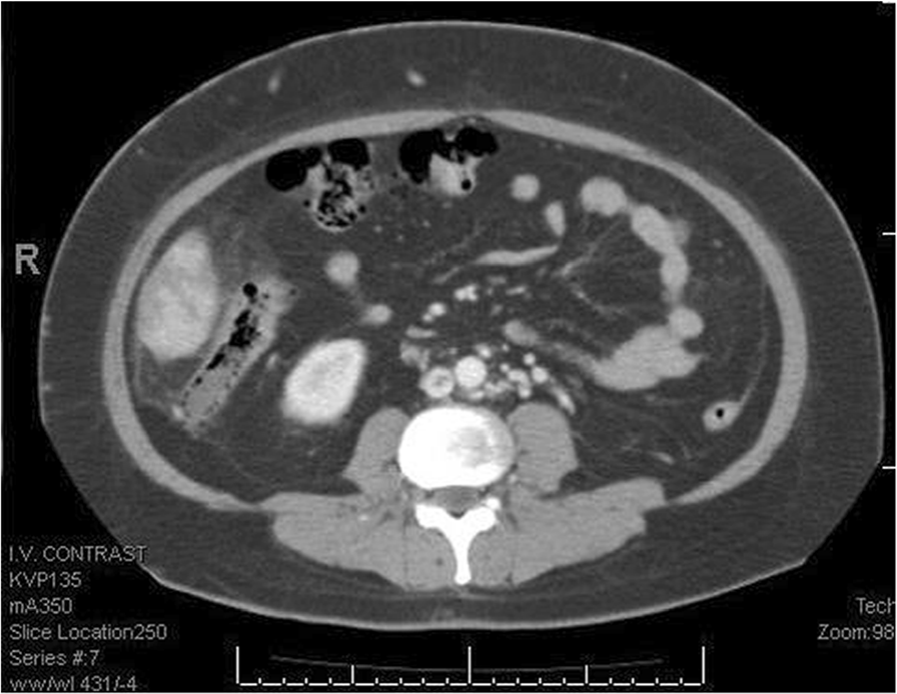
Computed tomography scan of the abdomen showing a clot within the inferior vena cava.
